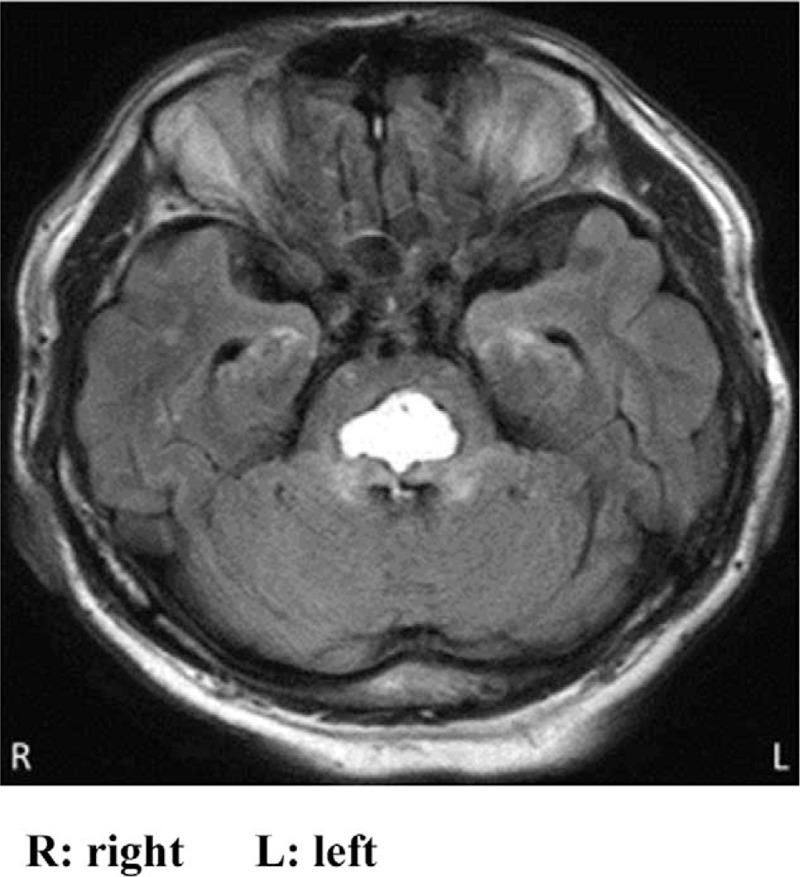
The axial image of the initial brain magnetic resonance imaging (T2 FLAIR) showed pontine hemorrhage with peripheral edema. FLAIR = fluid-attenuated inversion recovery.
